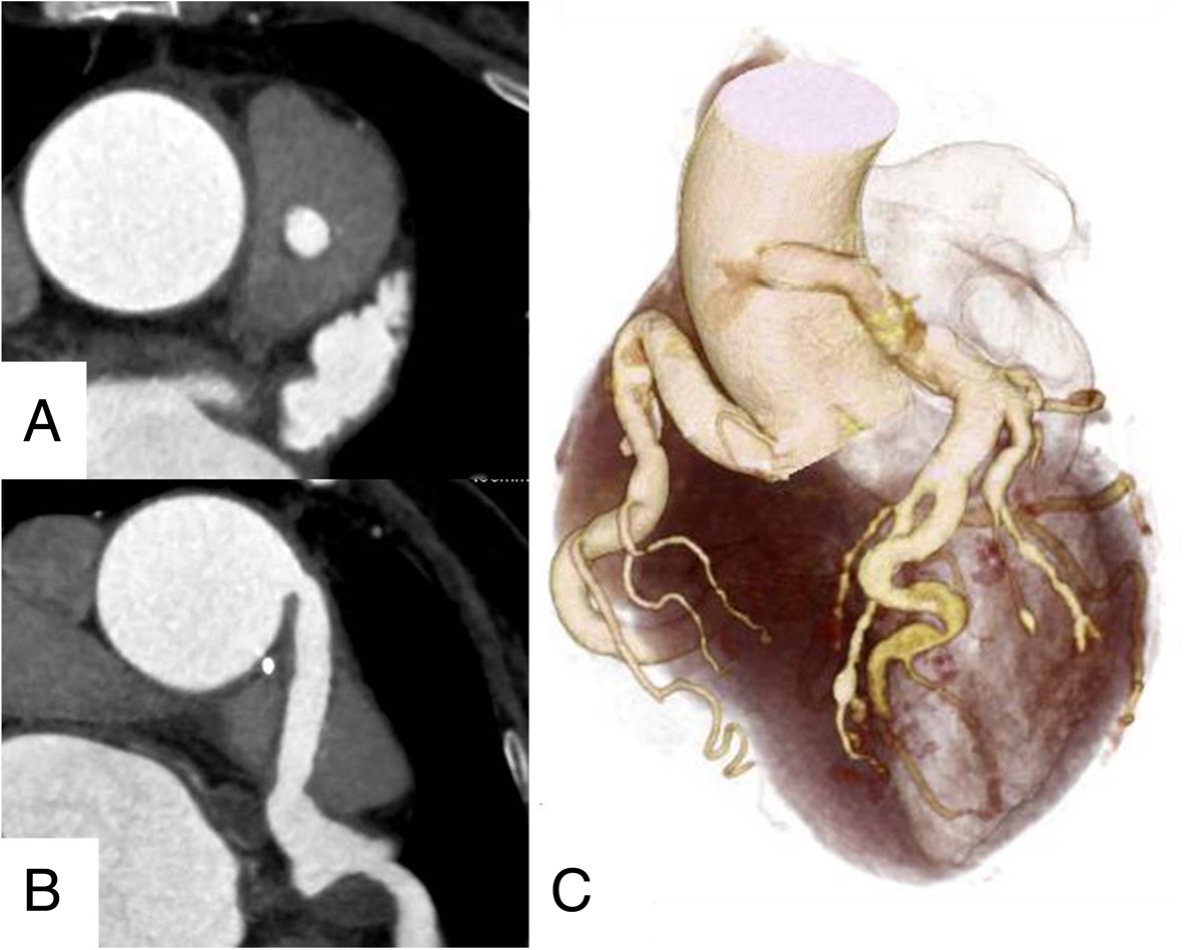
Post-operative computed tomographic image. a, b Postoperative computed tomography scan revealed the patency of the vascular prosthesis implanted in the main pulmonary artery at 2 year. The main pulmonary artery is also sufficiently patent. c 3D computed tomographic image revealed the vascular prosthesis penetrated through the main pulmonary artery
